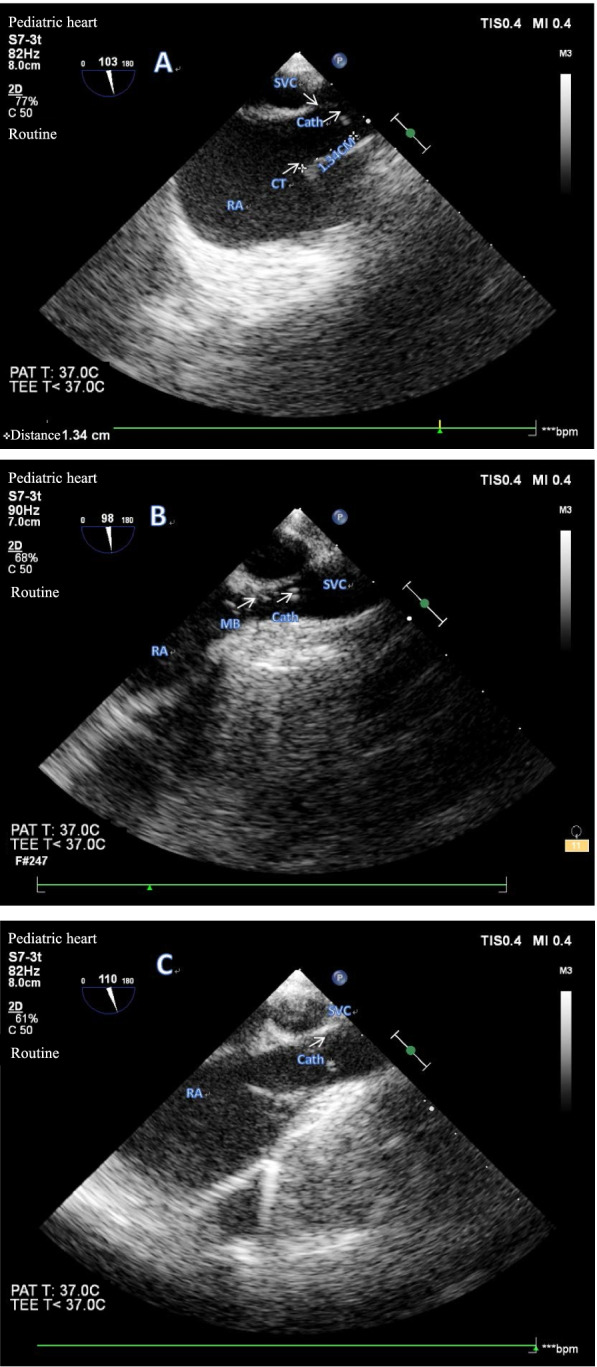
A TEE image of the catheter tip finally positioned, directly visualizing the end of the venous catheter 13.4 mm above the crista terminalis. B Multiple hyperechogenic microbubbles flowing quickly out of the distal catheter after a rapid flush of saline, confirming the location of the catheter tip. C Final confirmation of the location of the catheter tip using TEE after the catheter was connected to the port. SVC, superior vena cava; RA, right atrium; PAT, patient; TEE, transesophageal echocardiography
